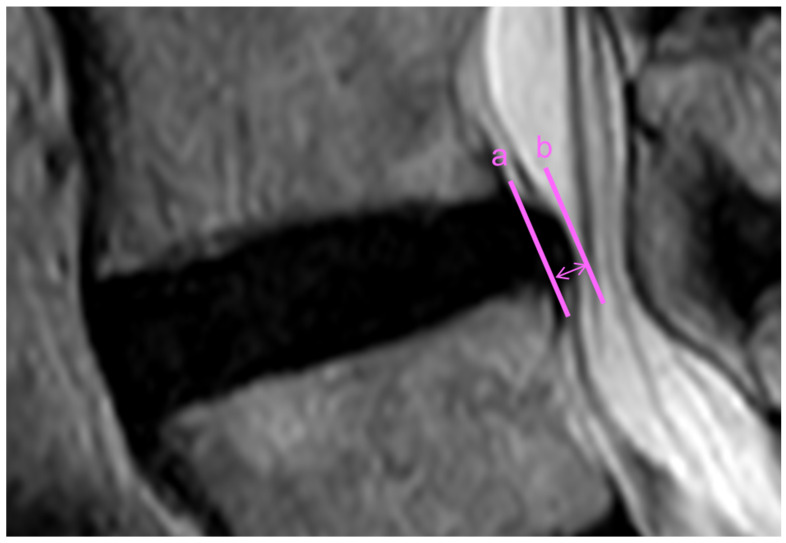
Measurement of disc bulging length (DBL). DBL (two-headed arrow), defined as the distance from the posterior end of the bulging disc (line a) to the posterior wall of the lumbar vertebra (line b), is measured on midsagittal magnetic resonance imaging (T2-weighted image).
